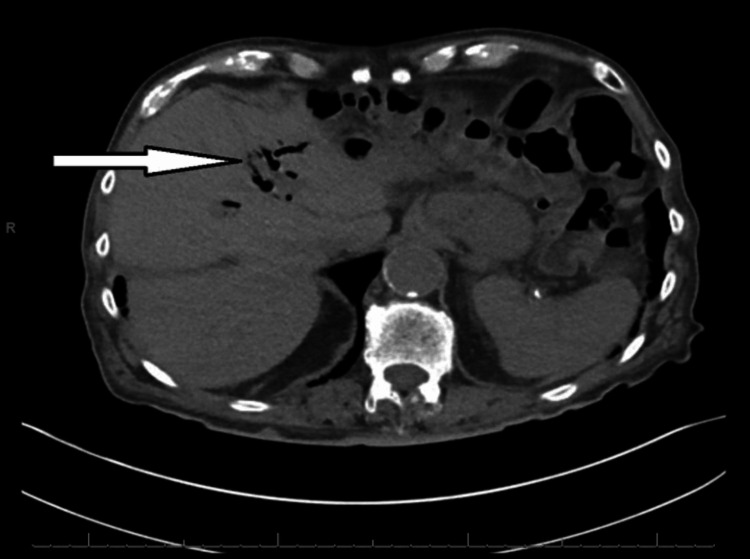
Axial plane of CT abdomen and pelvis two years prior to presentation with cholangitis and subsequent ERCP revealing pneumobilia (arrow).
